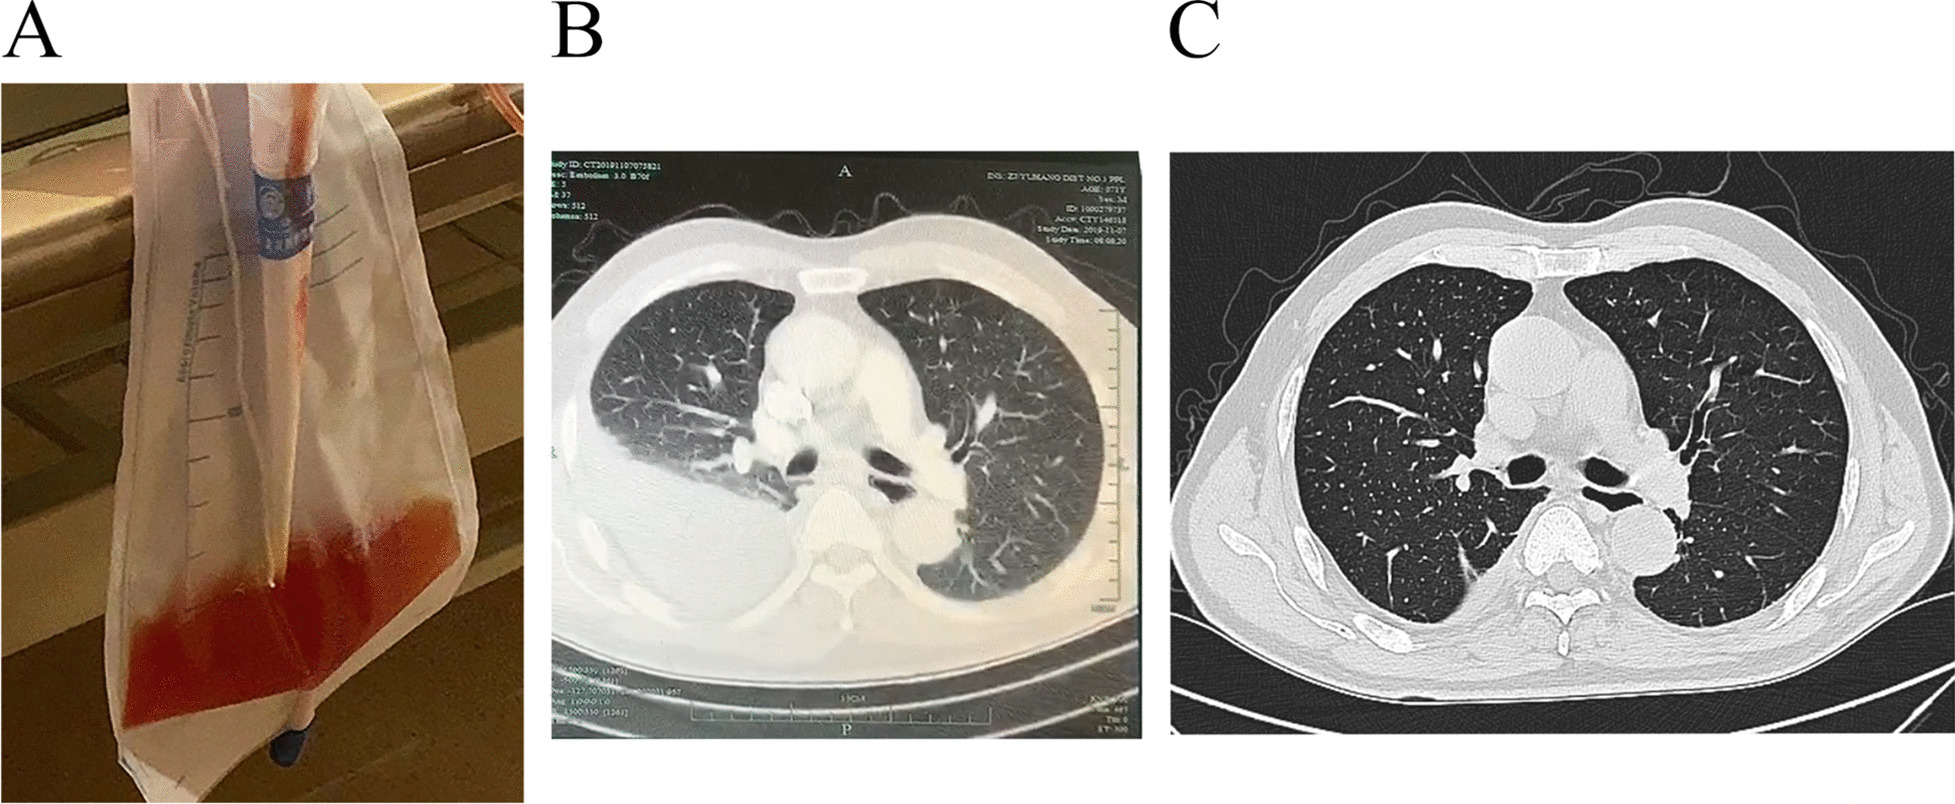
The appearance and CT scan of pleural effusion. A Appearance of the pleural effusion. B Chest CT showed moderate, right-sided pleural effusion on the day of admission. C Chest CT showed significantly reduced, small amount of right-sided pleural effusion 7 days after the admission.
